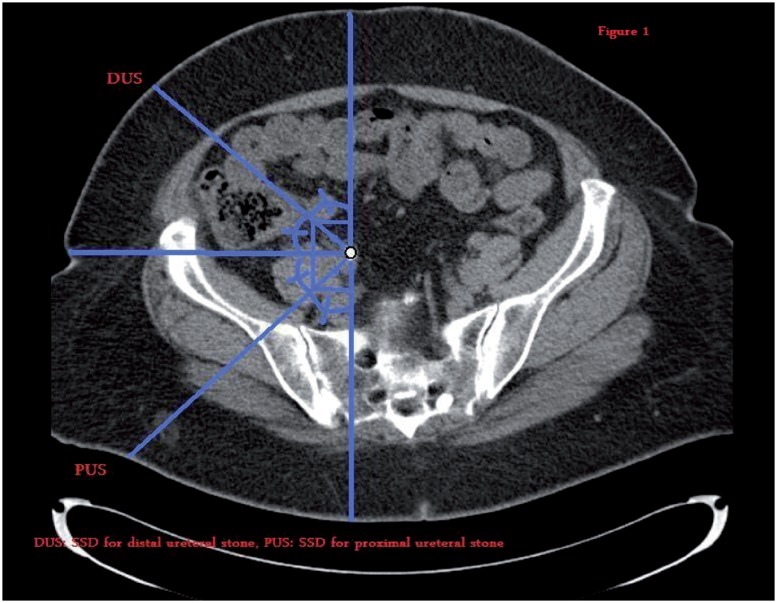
Showing pathways for ultrasonic waves.
